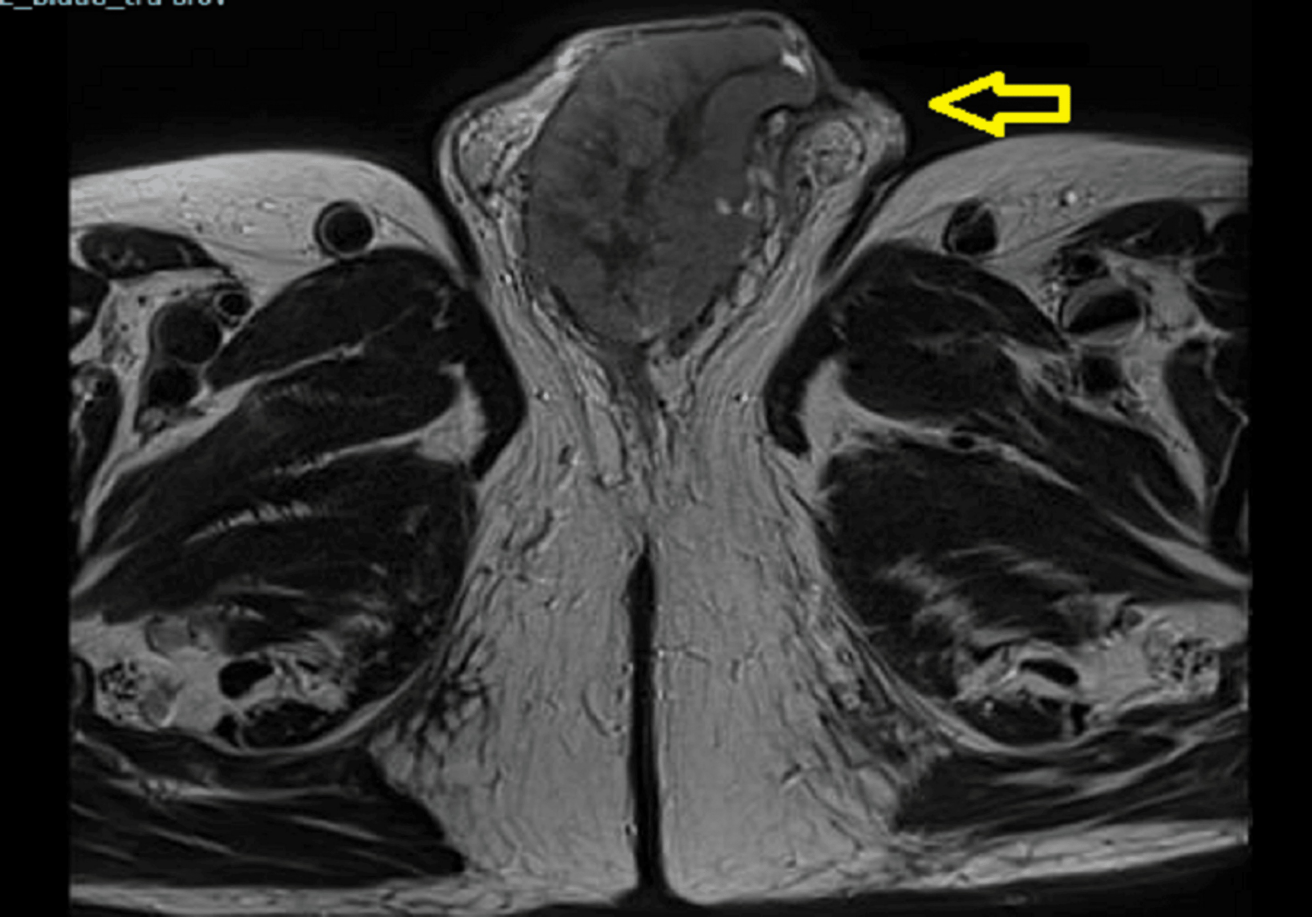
T2-weighted MRI of the pelvis showing a large penis (73.2 mm x 47.2 mm) poorly enhancing encapsulated mass within the inferior margin of the corpus spongiosum
